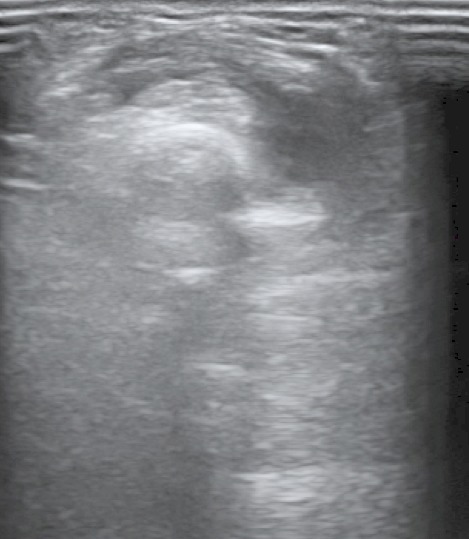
Ultrasonography wrist: axial section showing a peritendinous edematous thickening at the intersection of radial extensor tendons (ECRB, ECRL) with extensor pollicis longus (EPL)
